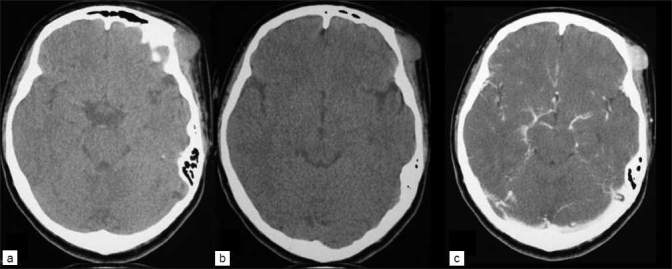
CT scan showing (a, b) precontrast images of extracranial mass of cutaneous malignant melanoma of the left supraorbital area with no bone involvement and (c) strongly enhanced melanoma
